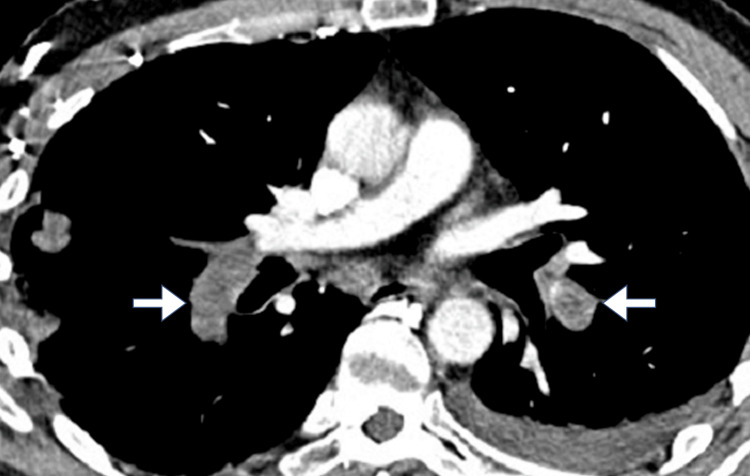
Axial CT pulmonary angiography image It demonstrates bilateral filling defects (arrow) consistent with acute pulmonary embolism.
